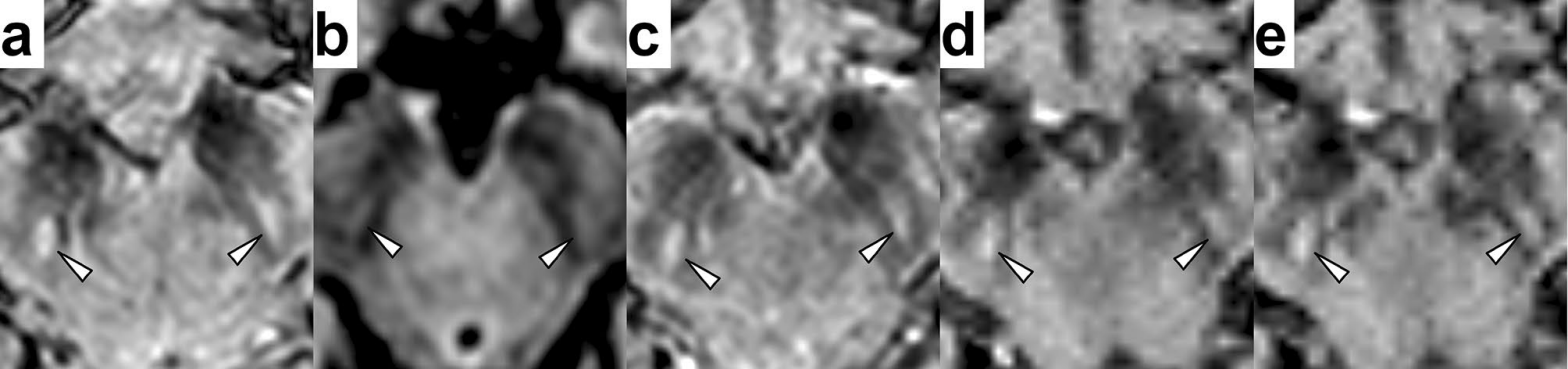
True-negative interpretation of the images of a 63-year-old healthy female subject. Nigral hyperintensity was bilaterally rated as “clearly present” (white arrowheads) on EPISEG (a), FLAIR (b), MEDIC (c), MAG (d), and SWI (e) images as well. Images are shown in radiological convention (right = subject’s left) at a level below the red nucleus.
